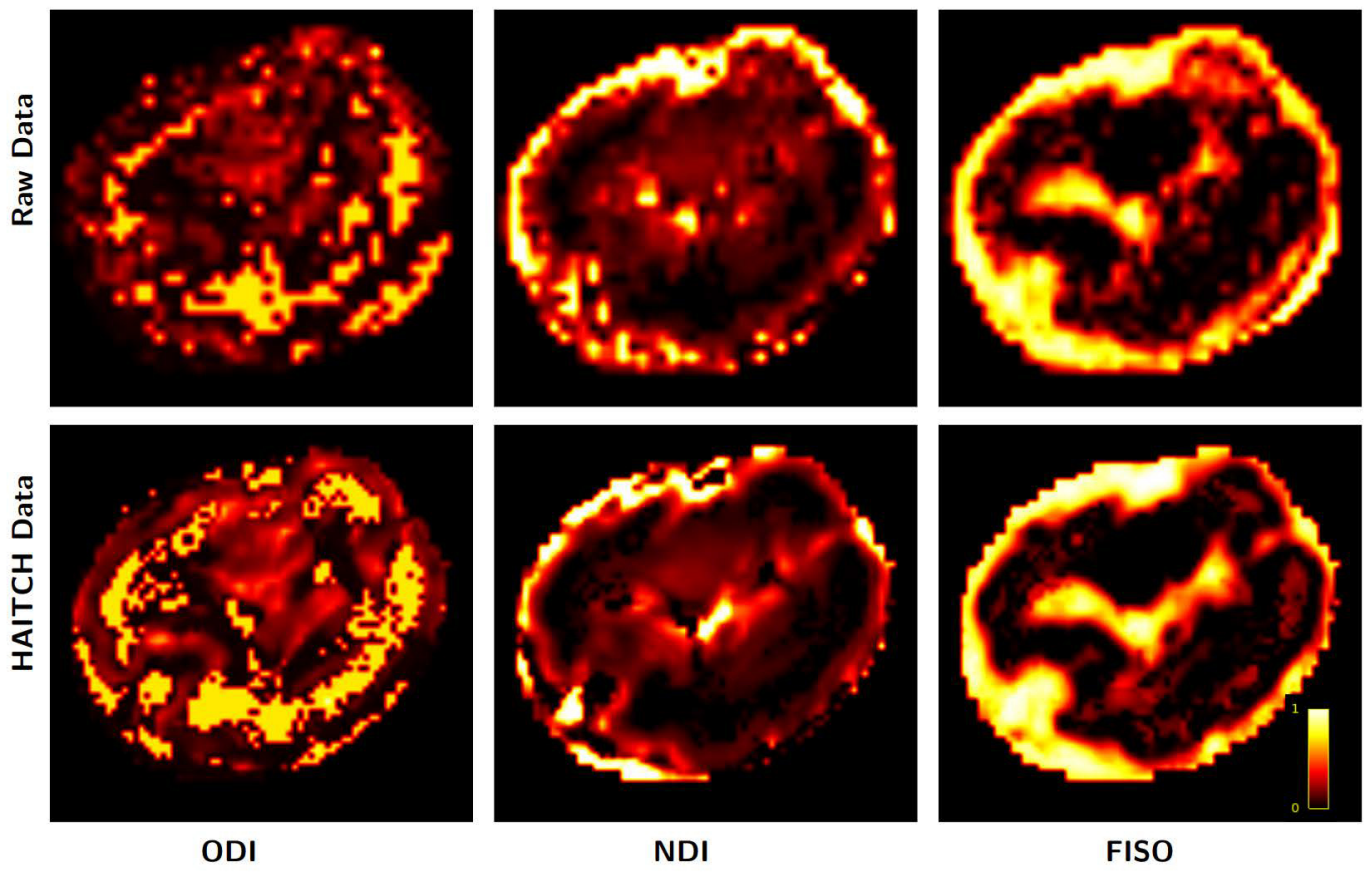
Comparison of NODDI parameter maps before and after applying the HAITCH correction. The top row shows the raw NODDI maps derived from uncorrected diffusion MRI data, while the bottom row shows the maps after motion and distortion correction using HAITCH. From left to right: Orientation Dispersion Index (ODI), Neurite Density Index (NDI, also referred to intracellular volume fraction - ICVF), and Free Water Volume Fraction (FISO). Detailed discussion of these results is provided inSection 5.
